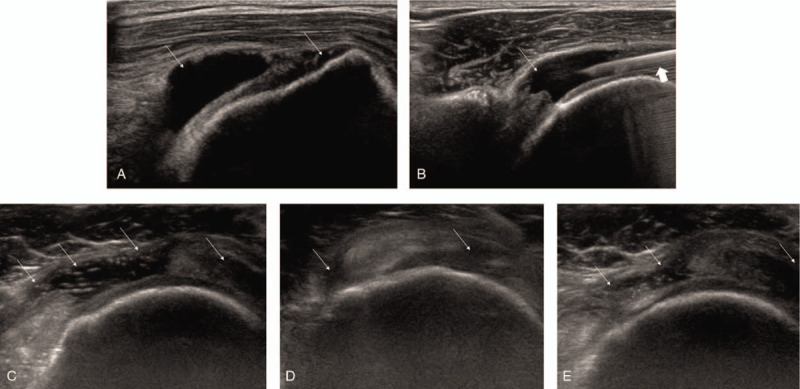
Ultrasound (US) revealing anechoic fluid collection (*thin arrow*) of subacromial-subdeltoid (SASD) bursa (A) and needle placement (*thick arrow*) within the SASD bursa (B). US revealing a large amount of anechoic fluid collection (*thin arrow*) and synovial hypertrophy of SASD bursa (C). Follow-up US 1 month later revealing diminution of the previously noted anechoic fluid (D). Follow-up US 2 month later revealing increased anechoic fluid compared to the previous US at 1 month; however, the fluid collection is slightly decreased compared to that noted in the initial US (E).
